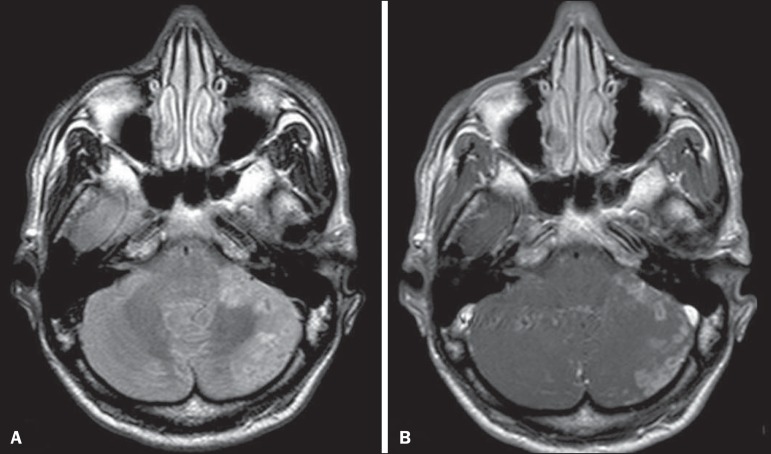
Tuberculosis. A: Axial FLAIR MRI sequence showing
